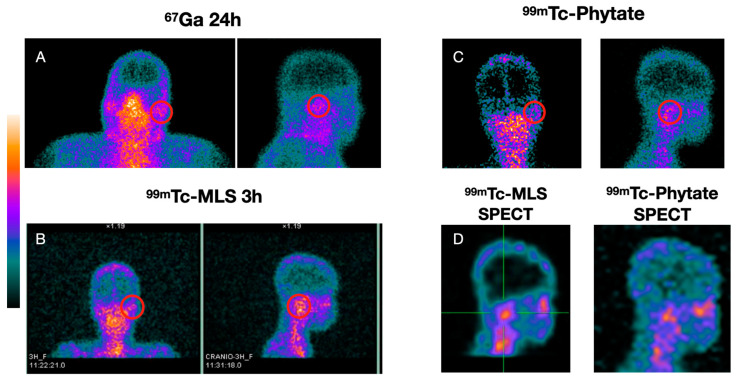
Patient 6. A male, 21 year-old patient with acute myeloid leukemia in remission with NEO suspicion in his right ear. Returning lab results revealed *Pseudomonas aeruginosa* positive culture, although no improvement was found after antibiotics treatment. Bone ^99m^Tc-MDP and ^67^Ga scintigraphy (A) were positive at the right mastoid topography. Both findings are in agreement with the infectious–inflammatory process in the temporal bone hypothesis. High uptake in ^99m^Tc-MLS in right mastoid topography (posterior and lateral incidence) 1 and 3 h (B) after labeled cells injection and enhanced with ^99m^Tc-phytate showing uptake in the same topography (C). SPECT (D) from ^99m^Tc-MLS and ^99m^Tc-phytate revealing the agreement in the uptake putting aside the infectious process possibility. After 6 weeks of antibiotics, a neoplasm was considered, and a biopsy revealed a myeloid sarcoma.
